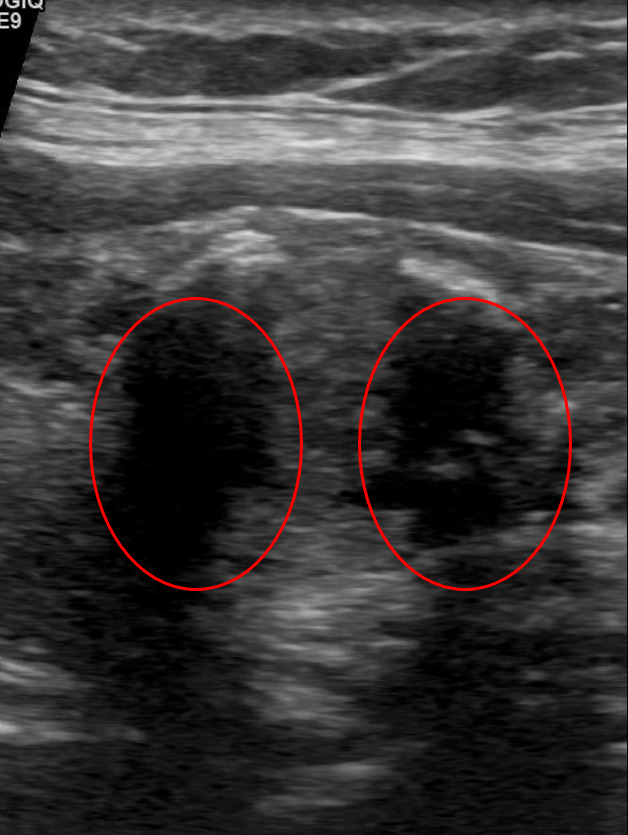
Ultrasound image from case 3 in the original dataset, showing a nodule with partial rim calcifications and consequent posterior acoustic shadowing (red circles). The nodule composition was erroneously classified as cystic (0 points) or mixed solid and cystic (1 point) in 19 out of 24 outputs, as opposed to almost completely solid (2 points) by the human rater.
